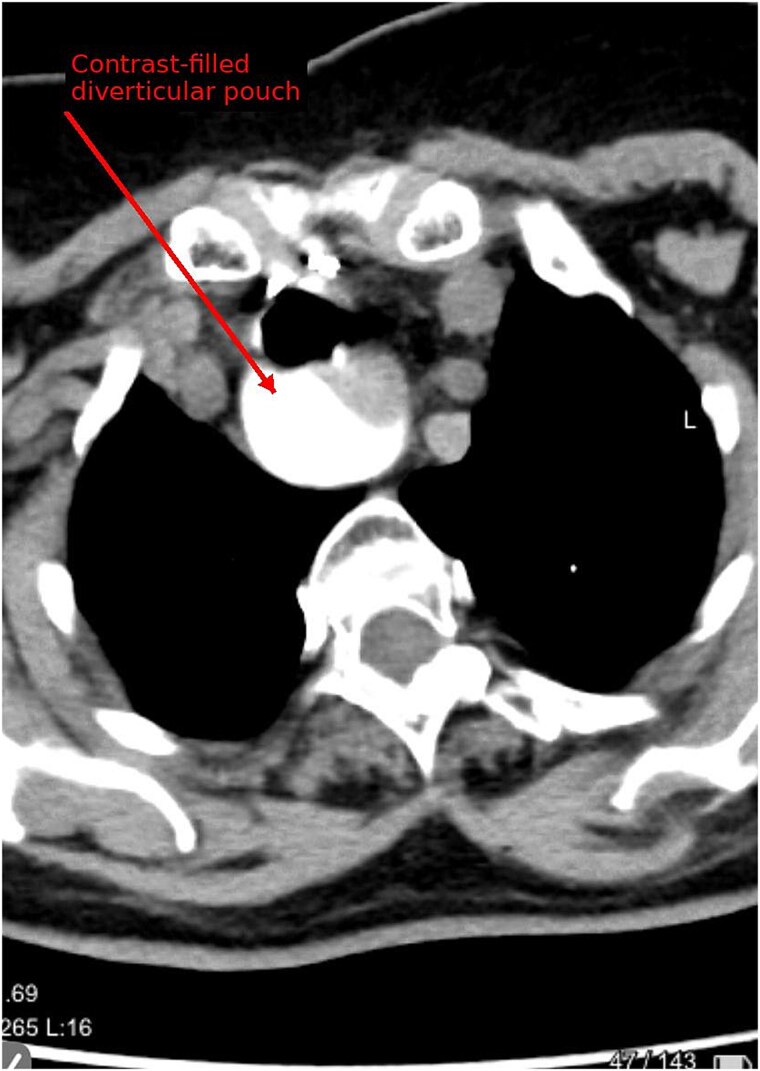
Contrast-enhanced CT (axial) demonstrating a contrast-opacified posterior pharyngoesophageal outpouching consistent with Zenker’s diverticulum.
